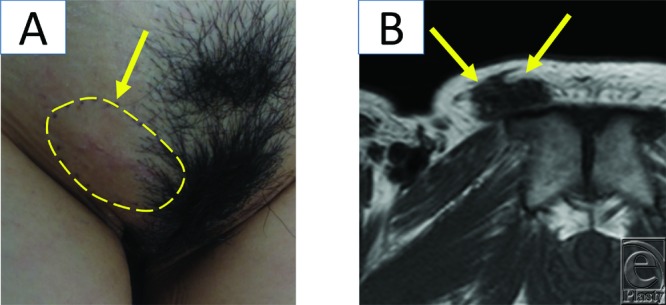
(*a*) Preoperative findings. A 41-year-old woman with an elastic, hard, and immobile 4 × 3.5-mm mass on the right part of the mons pubis. She had superficial pain on and around the tumor site. (*b*) Signal intensity of the tumor in the right inguinal region on magnetic resonance images. In this T1-weighted image, the tumor shows low signal intensity.
